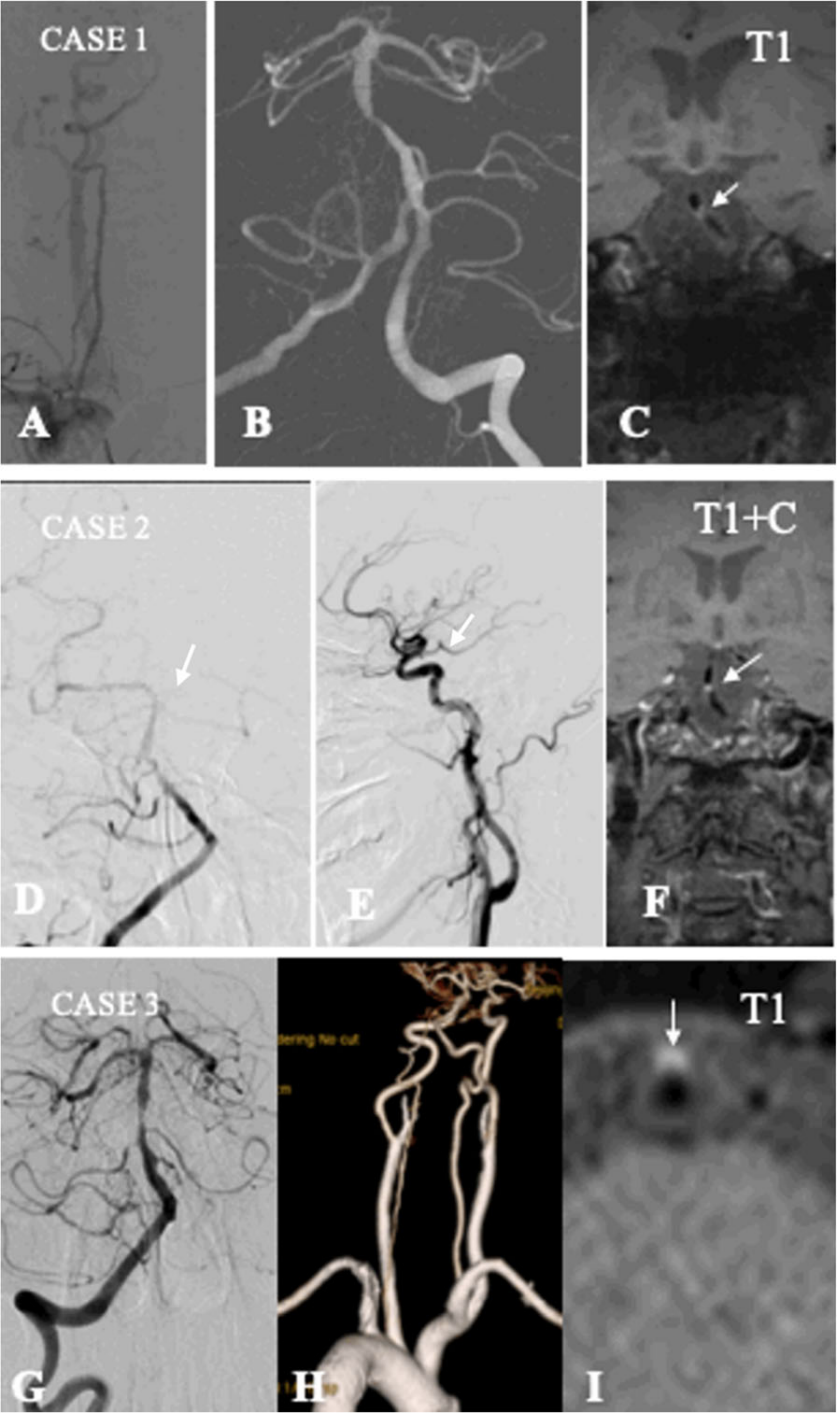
Example of complete and incomplete artery configuration of basilar artery. Case 1: the complete configuration of BA. a: the normal right VA with severe tortuosity of the first segment; b: normal left VA with severe stenosis of BA; c: HRMRI showing intraplaque hemorrhage of BA plaque on T1 weight imaging. Case 2: the incomplete distal configuration of BA. d: right VA angiography showing severe stenosis of BA without left PCA. e: left common carotid artery angiography showing fetal type PCA. f: HRMRI showing strong enhancement of BA plaque. Case 3: the incomplete proximal configuration of BA. G: dominant VA angiography showing severe stenosis of BA. H: CT angiography showing right VA hypoplasia. I: HRMRI showing intraplaque hemorrhage of BA plaque on T1 weight imaging
